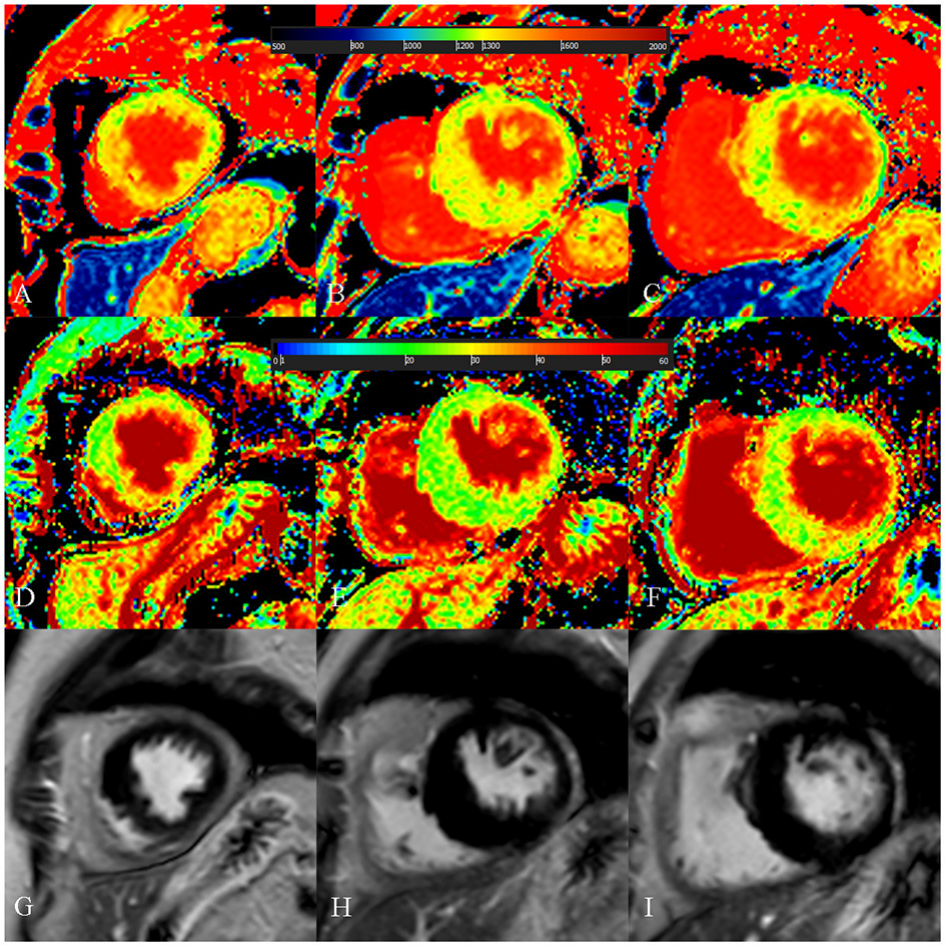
A 73-year-old female with focal midseptum hypertrophic cardiomyopathy. (A–C) Native T1 mapping of the apical, mid-, and basal portions of the left ventricular (LV) myocardium showed increased global native T1 (1,335.03 ms). (D–F) Extracellular volume fraction (ECV) of the apical, mid-, and basal portions of the LV myocardium showed normal global ECV (26.9%). (G–I) Late gadolinium enhancement (LGE) images of the apical, mid-, and basal portions of the LV myocardium showed no LGE. The maximal LV wall thickness was 19.8 mm, left atrial diameter was 40 mm, and the LV outflow tract gradient pressure was 95 mmHg. She had no family history of sudden cardiac death (SCD), non-sustained ventricular tachycardia, unexplained syncope, LGE/LV mass ≥15%, end-stage systolic dysfunction, and apical aneurysm. She was stratified as having low SCD risk under the 2014 European Society of Cardiology guidelines (5-year SCD probability: 1.70%) and enhanced American College of Cardiology/American Heart Association guidelines. However, she underwent cardiac-caused death 26 months after cardiac MRI examination. The elevated global native T1 mapping values could help indicate poor outcome in patients with low risk according to the two guidelines.
